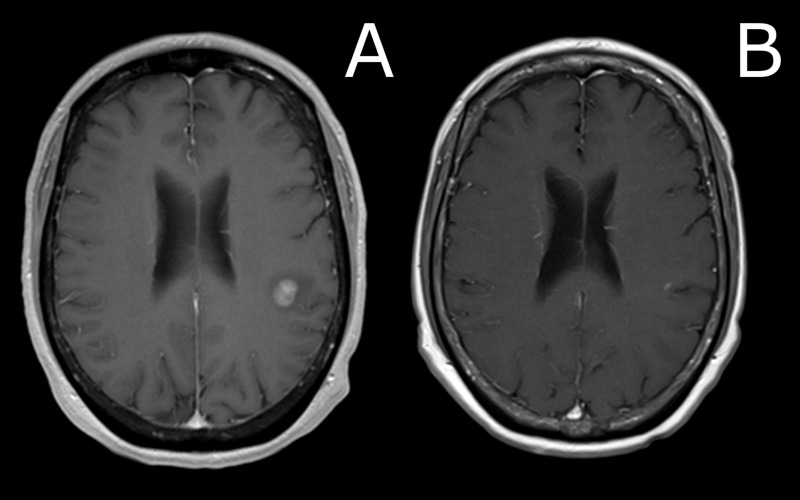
Comparison of brain MRI at presentation versus three months after SRS procedure A) MRI brain imaging revealed two contrast-enhancing lesions in the left frontal lobe (pictured in A); T1 axial image, post-gadolinium contrast) and left cerebellar hemisphere, measuring 1.25 cc and 0.07 cc, respectively. The patient underwent stereotactic radiosurgery (SRS), with 22 Gy delivered to the 50% isodose line to each lesion. B) Three months after SRS, repeat MRI demonstrated resolution of the frontal lobe lesion. MRI: magnetic resonance imaging
